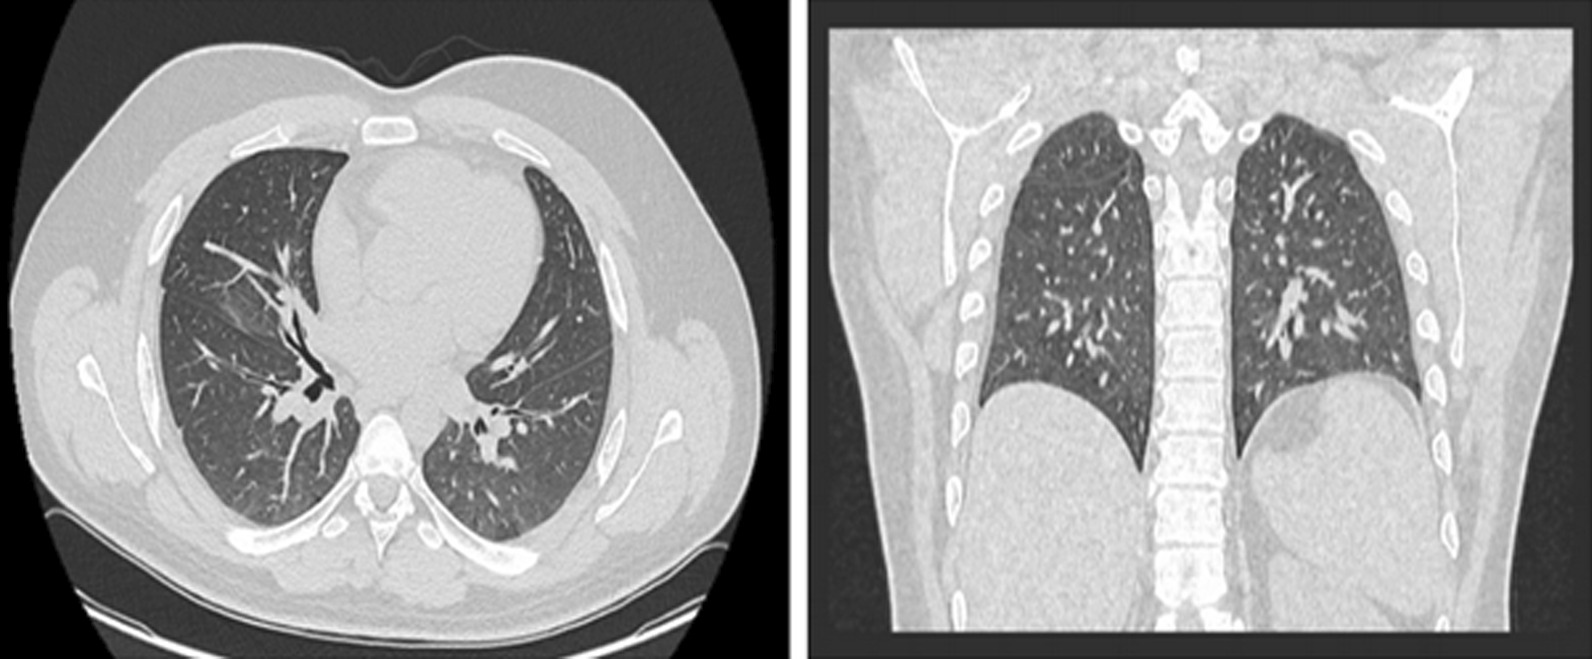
Transverse and coronal sections CT scan of the chest showing emphysematous changes
